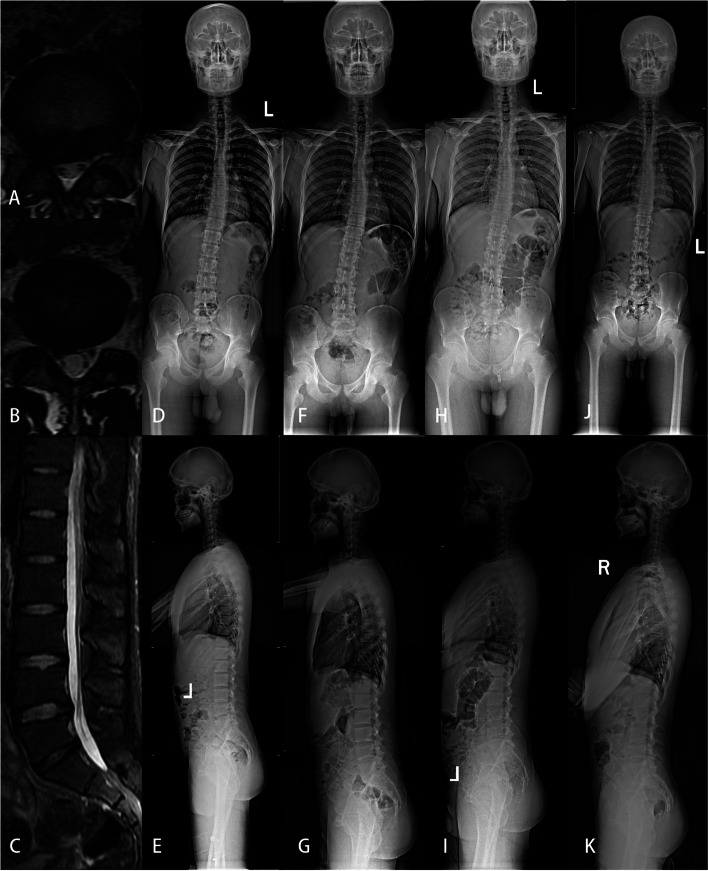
A 24-year-old male was admitted with “recurrent low back pain and right lower limb pain for more than one year”. He was diagnosed as lumbar disc herniation at L4/5 and L5/S1, and was treated with PELD in our hospital. A-E were preoperative lumbar magnetic resonance and whole spinal anteroposterior and lateral X-ray, A was L4/5 cross section, B was L5/S1 cross section, Cobb angle was 15.2 °; AVT:46.4 mm, CBD:54.2 mm, SVA:85.6 mm, TK:16.6 °, LL:41.4 °, PI:48.6 °; F-G were postoperative whole spinal positive and lateral X-ray, Cobb angle was 9.3°; AVT:45.4 mm, CBD:52.3 mm. SVA:84.1 mm, TK:17.7 °, LL:49.1 °; H-I: Cobb angle: 5.8 mm, AVT:39.7 mm, CBD: 40.5 mm, SV-A: 34.3 mm; TK: 17.8 mm, LL:49.4 °; J-K: Cobb angle: 3.9°, AVT:18.9 mm, CBD:28.7 mm, SVA:-2.2 mm, TK:20.1 °, LL:50.5 °
